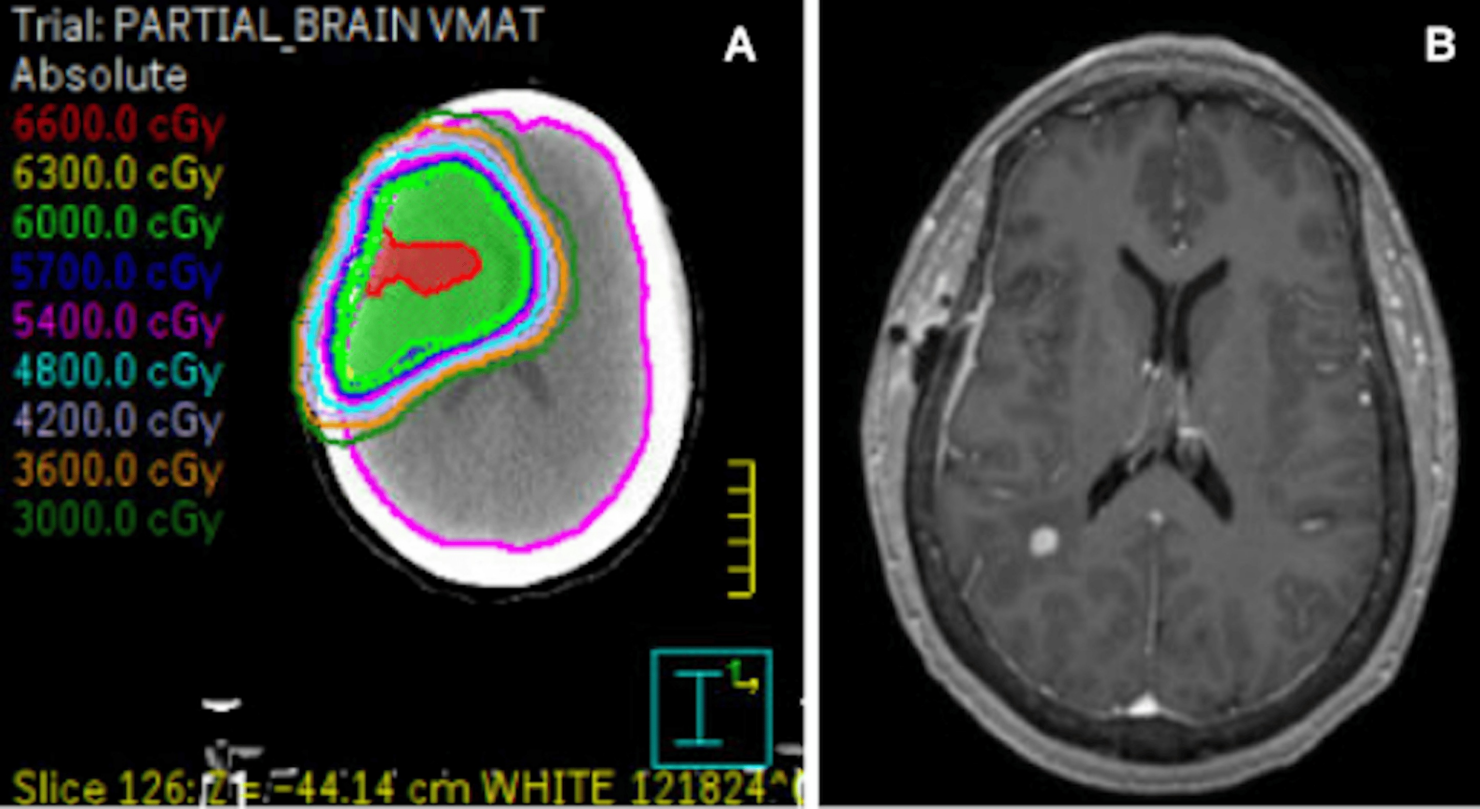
Prior radiation field (A) and tumor location on MRI (B).
